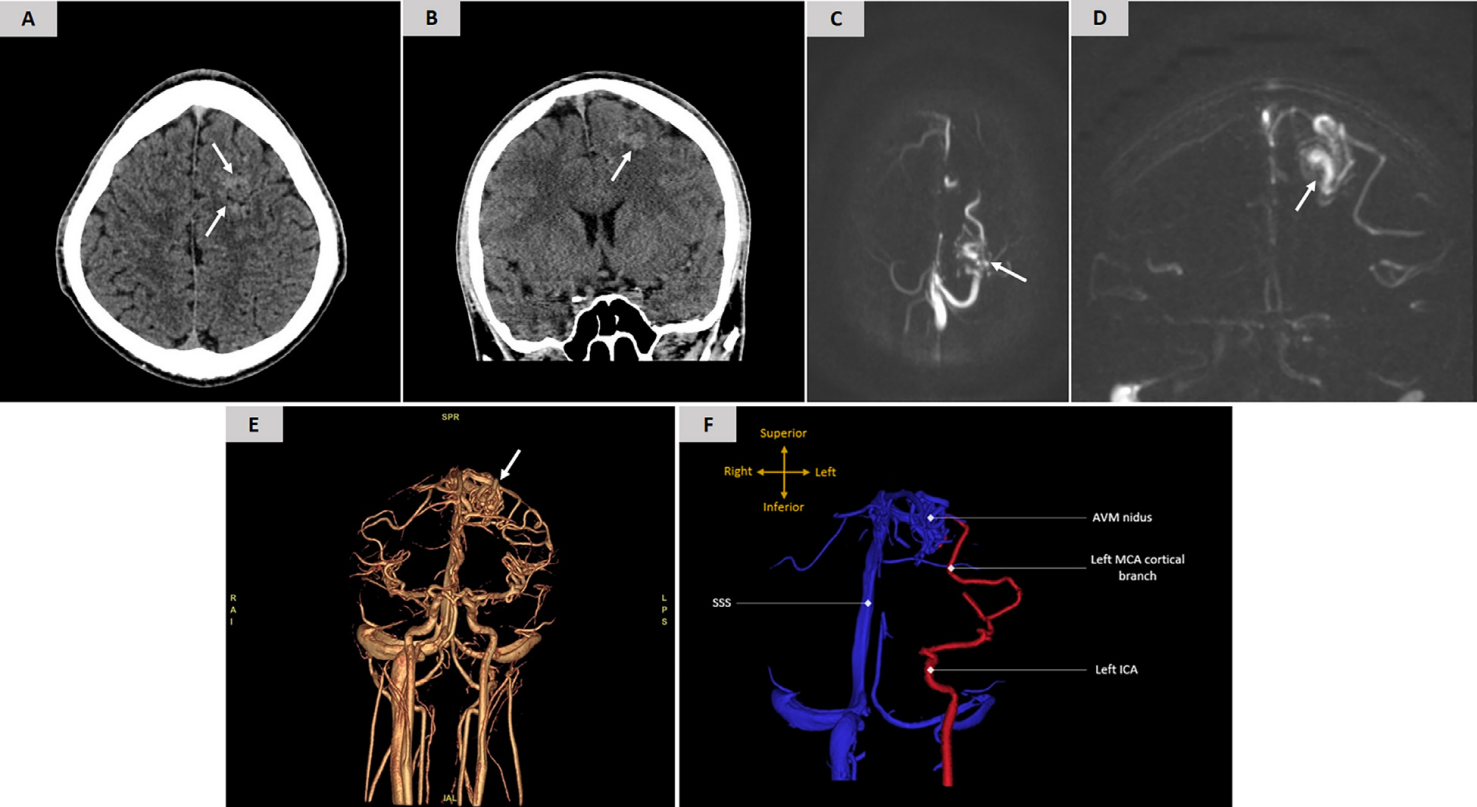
(A) Axial and (B) Coronal head CT images demonstrate a tuft of abnormal vessels located on the left superior frontal gyral surface (*arrows*). (C) Axial and (D) Coronal MR venogram images reveal a vascular nidus of approximately 2.0 × 2.0 cm involving the left superior surface of the frontal lobe (*arrows*). (E) VRT reconstruction image and (F) Annotated depiction of the arterial feeder, nidus, and venous drainage of the detected AVM lesion. (AVM, arteriovenous malformation, MCA, middle cerebral artery, SSS, superior sagittal sinus, ICA, internal carotid artery)
